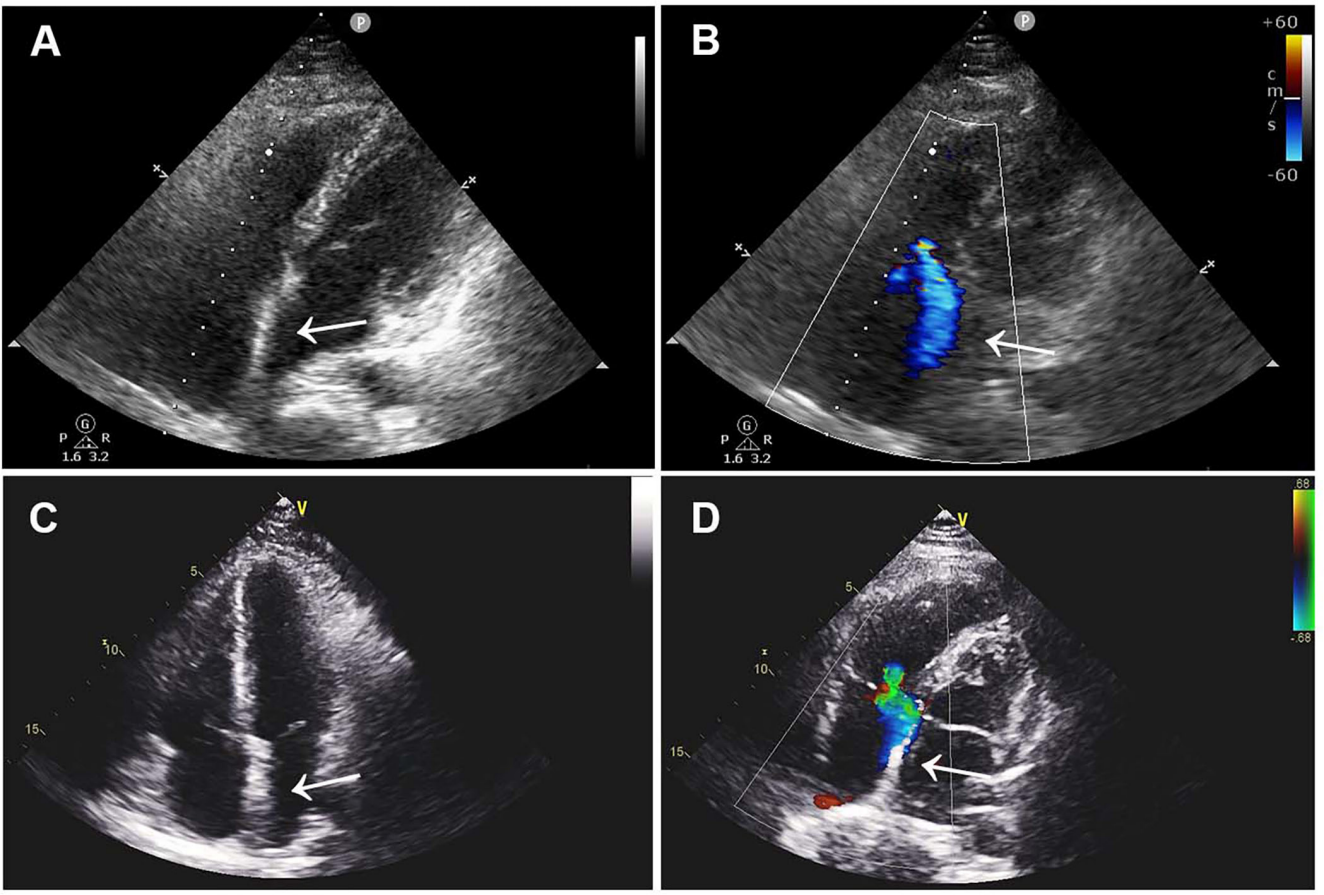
Postoperative TTE images. (A,B) TTE images 1 month after surgery showed continuous atrial septum without shunt and thrombus. (C,D) Three-month postoperative TTE images showed no novel shunt and no thrombus. TTE, transthoracic echocardiography.
